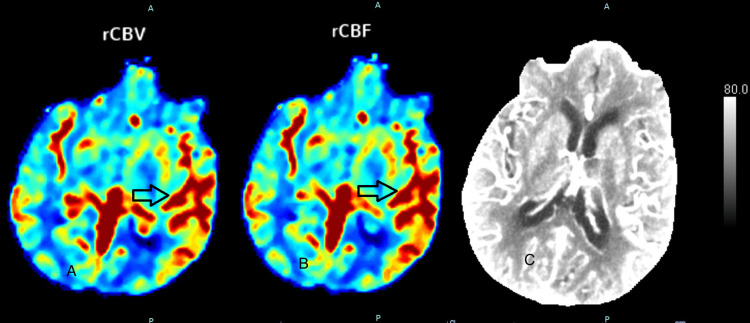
Brain CT perfusion scan (A) is  cerebral blood volume (CBV) and (B) is cerebral blood flow (CBF). Both CBV and CBF show increase in perfusion of the left temporal lobe without any corresponding vascular defect in (C).
